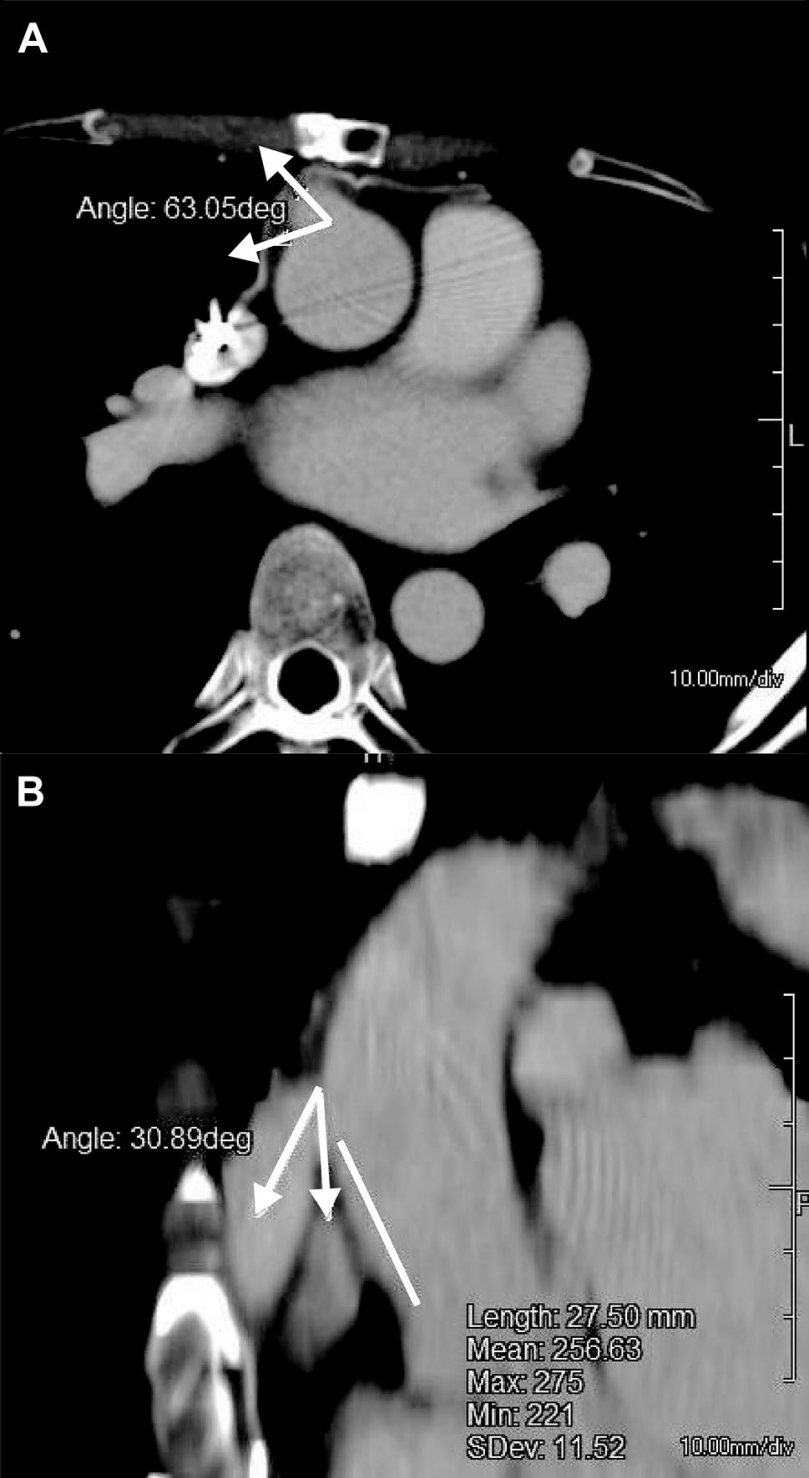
Representative computed tomographic images used to examine outflow graft anastomosis design. A Angle indicates azimuth angle. B Angle indicates inclination angle. Line indicates distance between sino-tubular junction and graft anastomosis location
